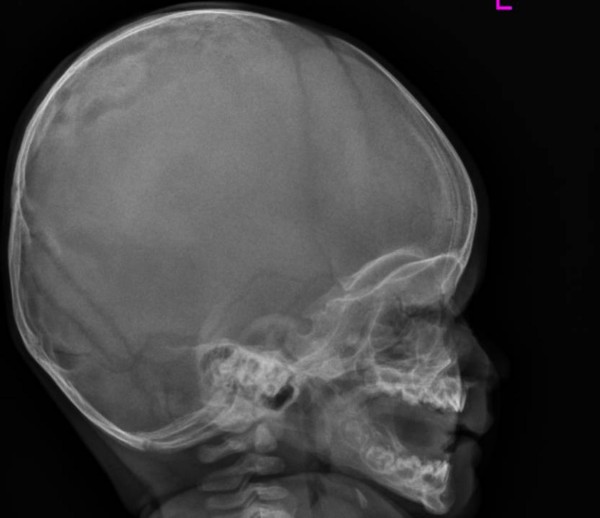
Lateral radiograph of the skull showed large osteolytic/multicystic lesion associated with thick sclerotic rind in the right parietal bone.
